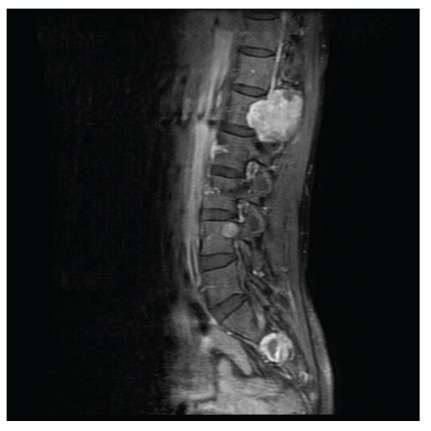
A T1-weighted MRI scan of the spinal cord showing two enhancing extradural hypointense EBV-SMT, approximately 3 cm and 1 cm in diameter, present at the right neural foramina of L3 and S1, respectively (image reproduced with permission from [13]. The AIDS Reader, UBM Medica).
